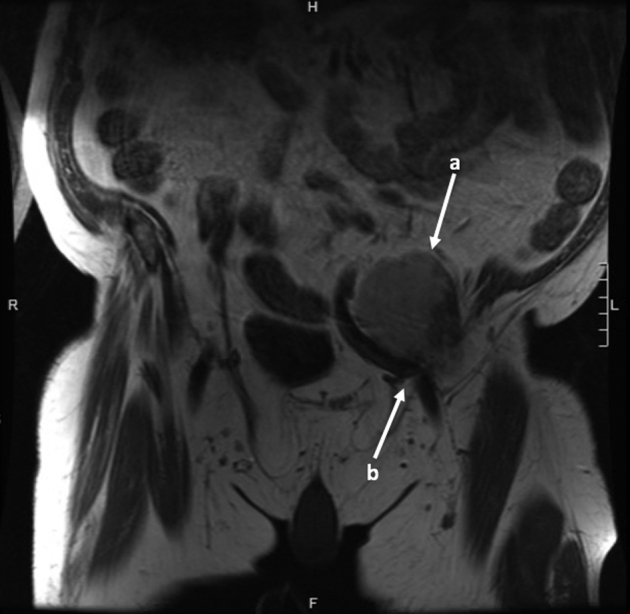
Metal artifact reduction sequence (MARS) magnetic resonance imaging (MRI) demonstrates a distinct anterior hip mass (a), emanating from the hip joint and encroaching on the anterior vascular structures (b).
